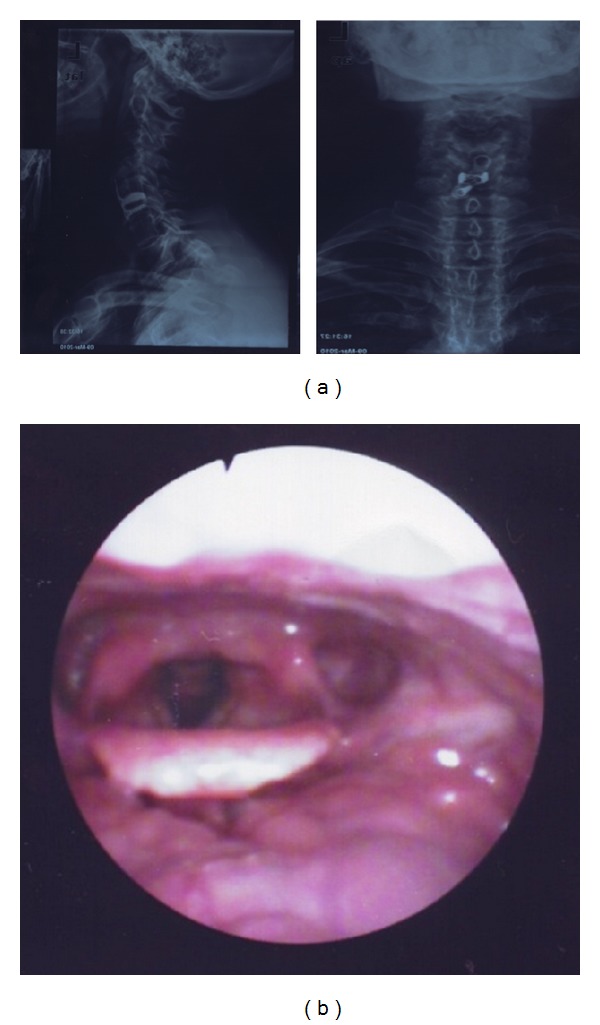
Radiographic and endoscopic controls after 3 years.
